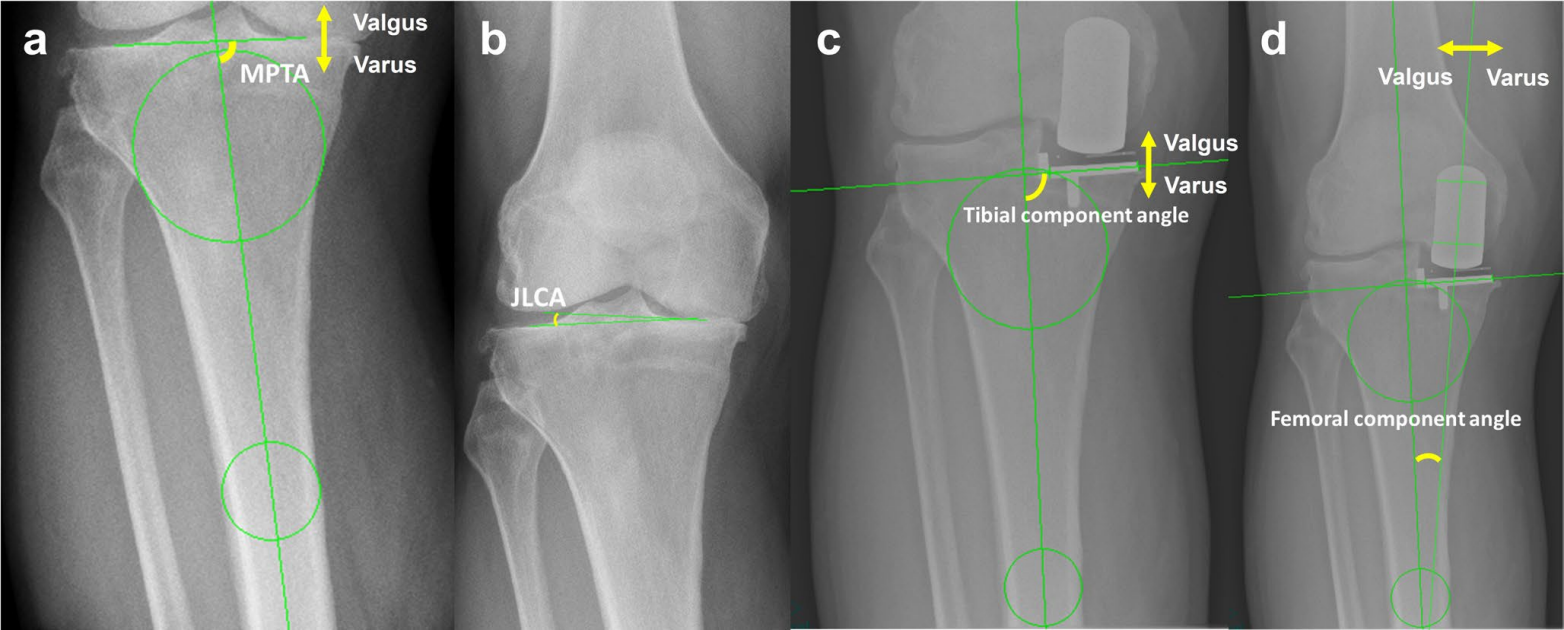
Radiographic measurement parameters. Representative radiographs showing the measurement methods. (a) Medial proximal tibial angle (MPTA): the angle between the tibial and tibial mechanical axes. (b) JLCA: joint line convergence angle. (c) Tibial component angle: angle between the tibial axis and the bisector line of the tibial component. (d) Femoral component angle: angle between the tibial axis and the bisector line of the femoral component. For consistency, valgus alignment on anteroposterior radiographs was defined as negative and varus alignment as positive
